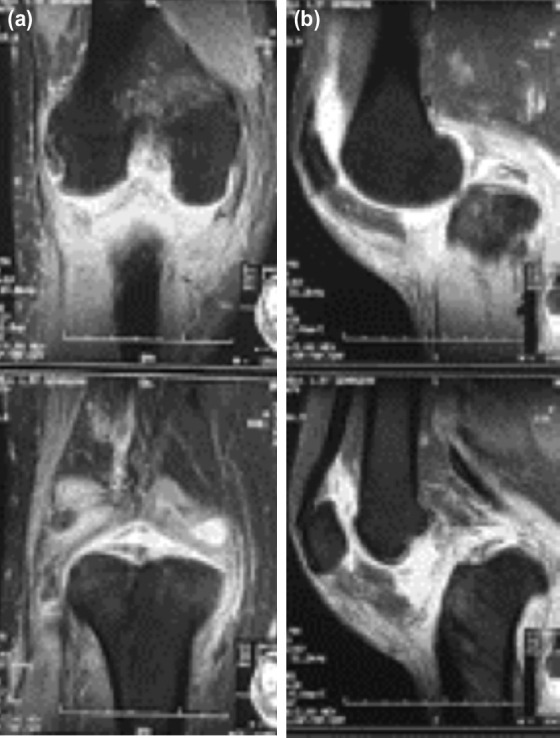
Magnetic resonance imaging of knee, (a) coronal view, (b) sagittal view.
